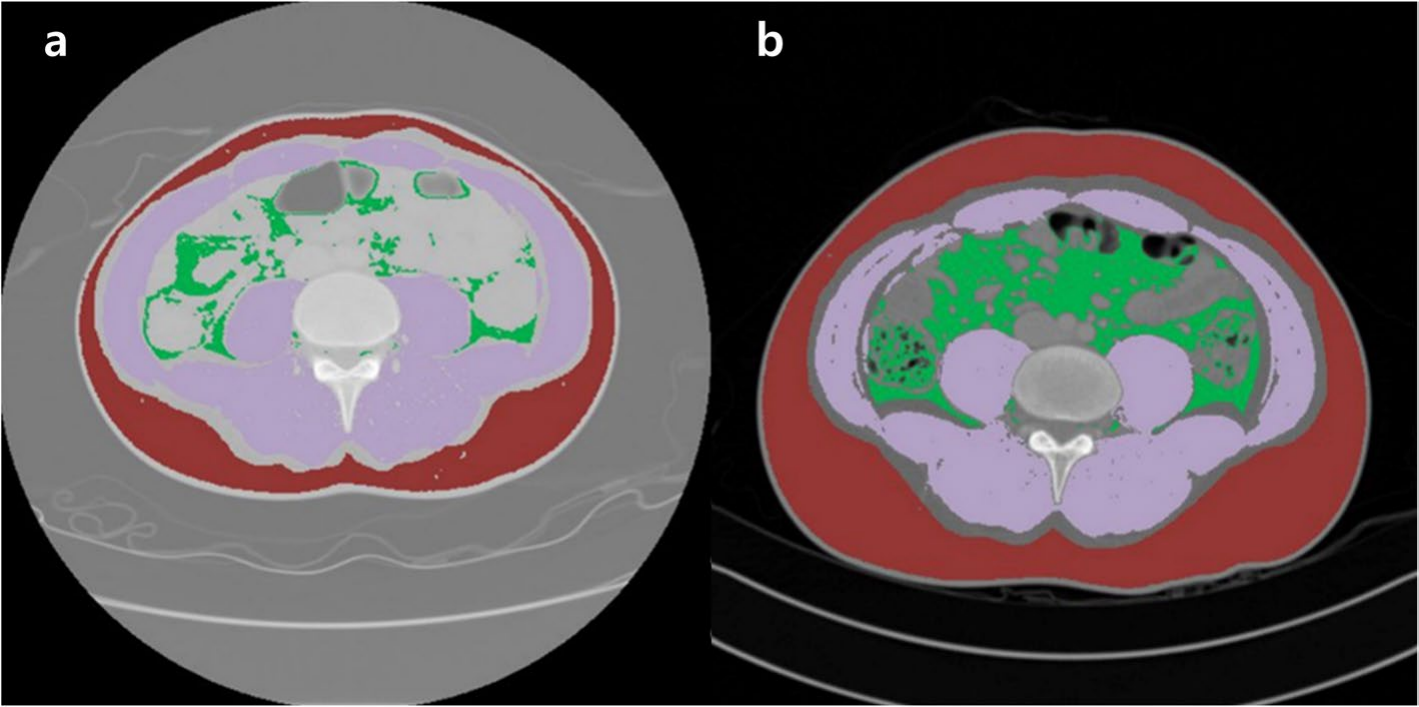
Evaluation of the body composition parameters at the L3 vertebral level using computed tomography scans. (a) Baseline (b) After treatment with biologic agents. Purple: skeletal muscle area (SMA), red: subcutaneous fat area (SFA), green: visceral fat area (VFA)
